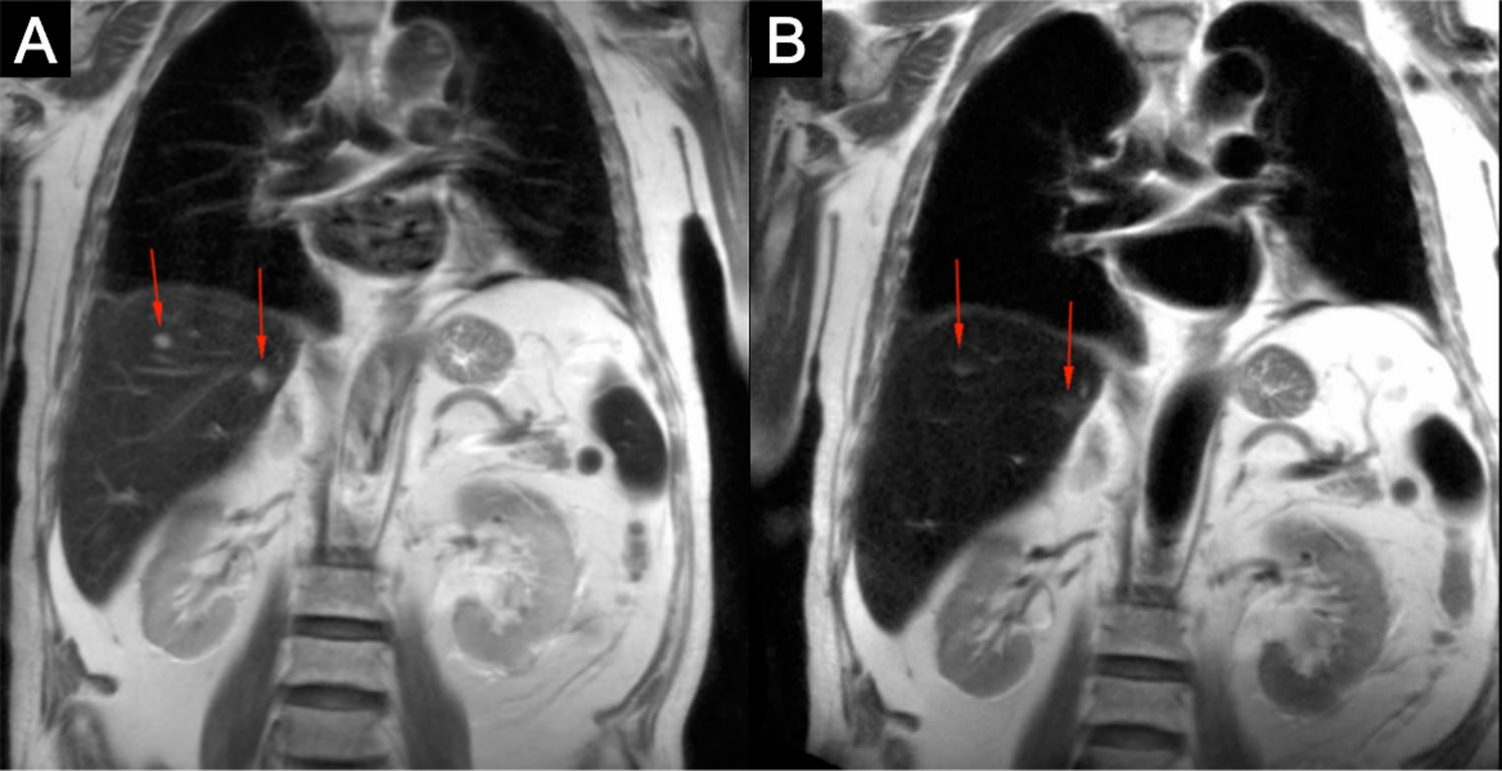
3.0 T ferumoxytol-enhanced MRI in a 74 year-old male with hemangiomas. Pre-contrast (a) T2 HASTE acquisitions show round hyperintense lesions (red arrows) indistinguishable from cysts without contrast. Post-contrast (b) T2 HASTE acquisitions show loss of signal for the lesions (red arrows) which is suggestive of hemangiomas
